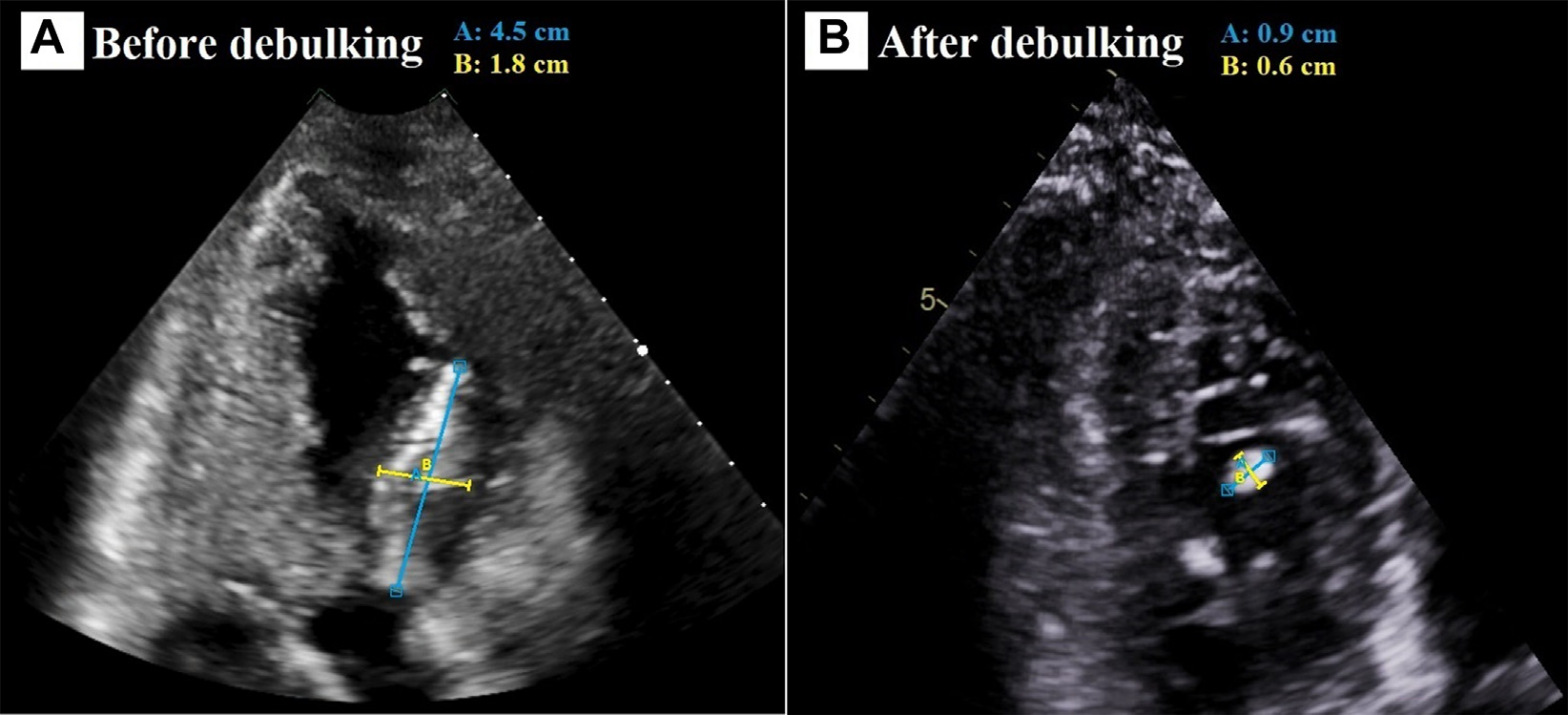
Echocardiogram in the Apical 2-Chamber View (Still Images) Demonstrating Successful Debulking of the Intracardiac Mass (A) Large intracardiac mass measuring 4.5 × 1.8 cm (before debulking). (B) Significant reduction of the intracardiac mass size to 0.9 × 0.6 cm (after debulking).
